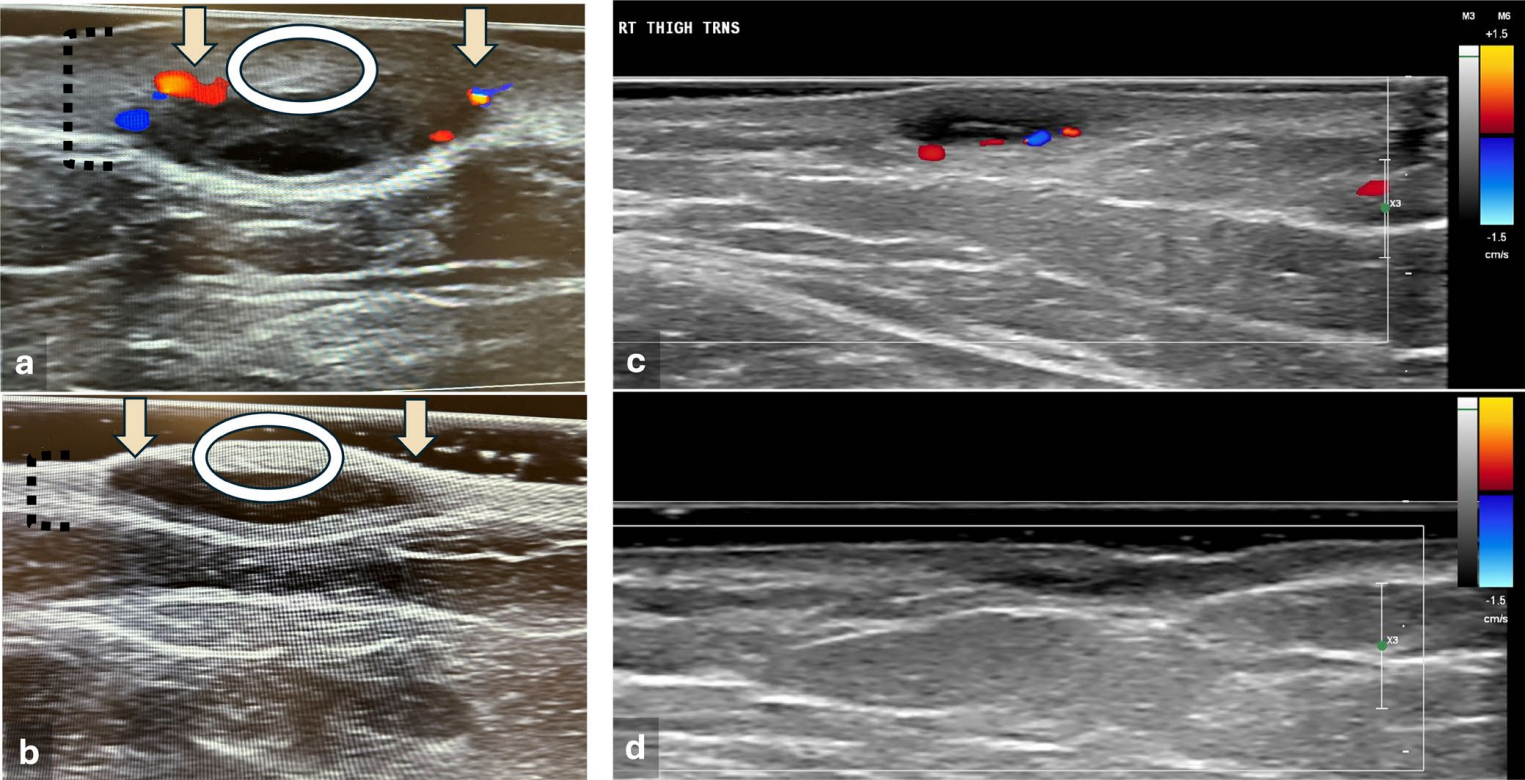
High‐frequency ultrasound evaluation before and after fractional microneedling radiofrequency (RF) treatment. (a–b) HFUS images of a dermal tunnel on the buttocks before treatment (a) and after five sessions of fractional microneedling RF (b), demonstrating reduced dermal thickness (dashed lines), increased echogenicity (circle), decreased tunnel size, and loss of vascular flow (arrows), consistent with resolution of active inflammation. (c–d) Type A dermal tunnel in the thigh with baseline findings of dermal thickening, reduced echogenicity, and a central hyperechoic line consistent with retained hair follicles. Color Doppler revealing hypervascularity at the tunnel base (c). After two treatment sessions (d), there is marked reduction in tunnel size, increased dermal echogenicity and thickness, and complete loss of vascular flow on Doppler imaging.
